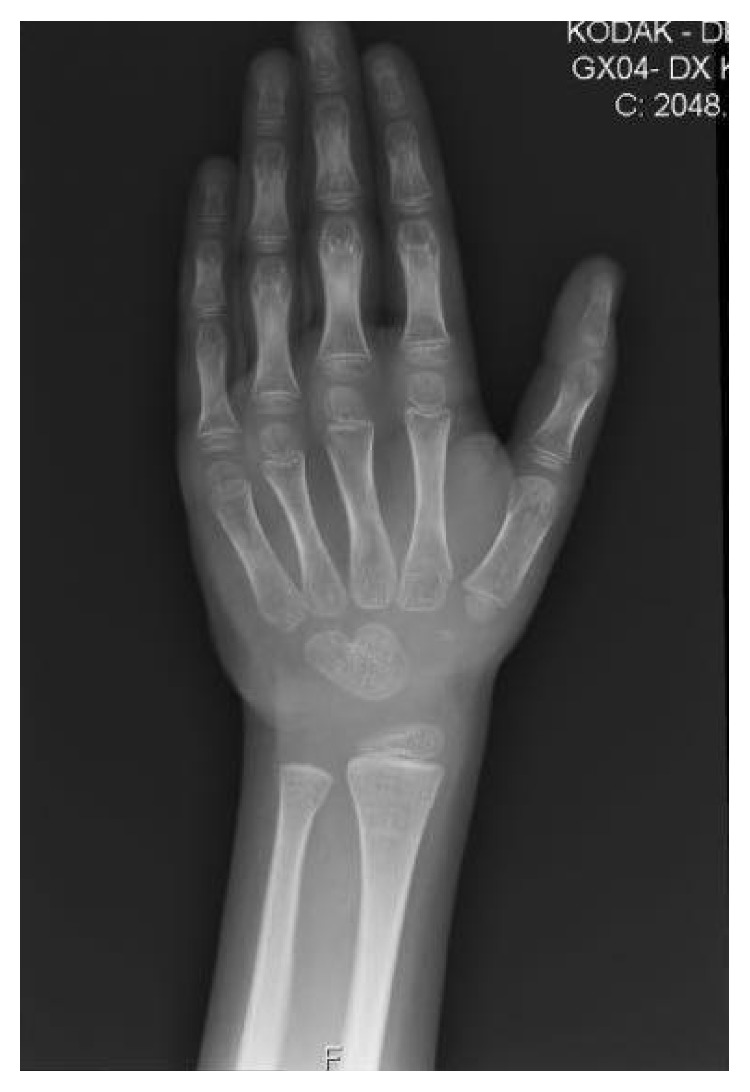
Significant osteopenia noted on left hand radiograph and a reduction in bone age of 1 year based on the metacarpal and phalangeal epiphysis.
